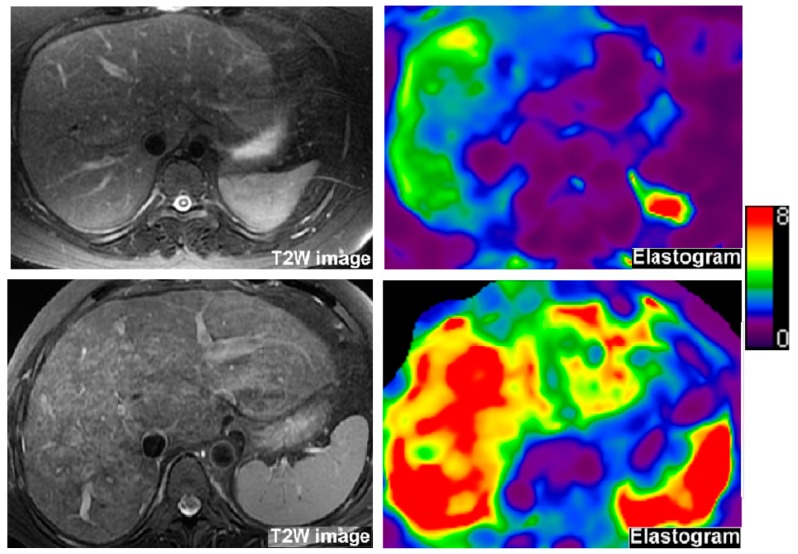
Magnetic resonance elastography images to measure liver stiffness in two patients with history of Fontan palliation. Left images: T2-weighted anatomic images. Right images: MR elastograms showing tissue stiffness. First row of images are from a 31 year-old female with no history of thromboembolism with liver stiffness of 3.0 kPa (upper limit of normal is below 2.5–2.7 kPa). Anatomic images show an enlarged, mildly T2-weighted hyperintense liver compatible with congestion and possible mild fibrosis. The elastogram shows mildly elevated liver stiffness (green and yellow on the color map) with the color scale provided for reference. Second row of images are from a 42-year-old female Fontan patient with liver stiffness of 6.4 kPa. She had atriopulmonary Fontan and a history of bilateral pulmonary embolus due to a right atrial thrombus. Anatomic images show an enlarged liver with subtle surface nodularity and reticular increased T2-weighted signal suggestive of substantial fibrosis in addition to congestion (the hepatic veins appear engorged). There is splenomegaly suggesting portal hypertension. The elastogram shows diffusely increased liver stiffness (e.g., areas of red color).
